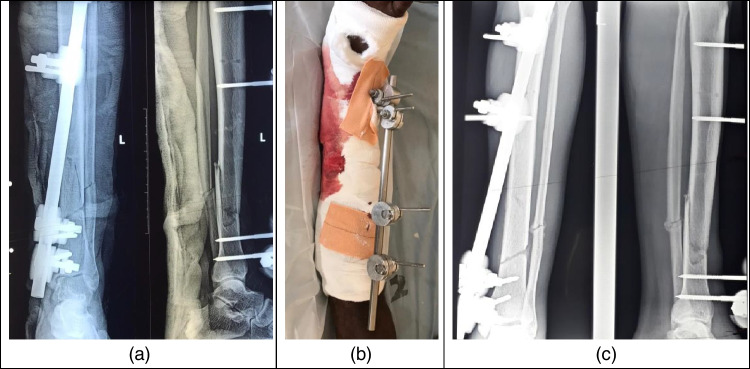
Case study of the external fixator’s unilateral uniplanar configuration. a Initial radiographs. b External view of initial fixation. c Radiographs at 2 weeks
